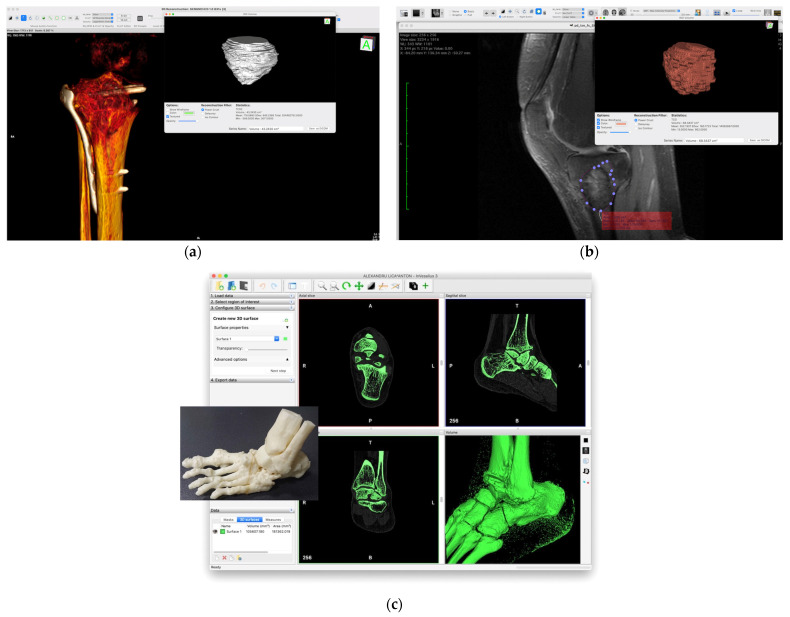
Patient-adapted analysis based on 3D medical image segmentation software using anonymized DICOM images: definition of bone defects volume/topography on (a) CT—image; (b) MRI image with identification of the bone defect (purple dot circle); (c) Patient leg reconstruction (3D-printed model for surgical planning).
